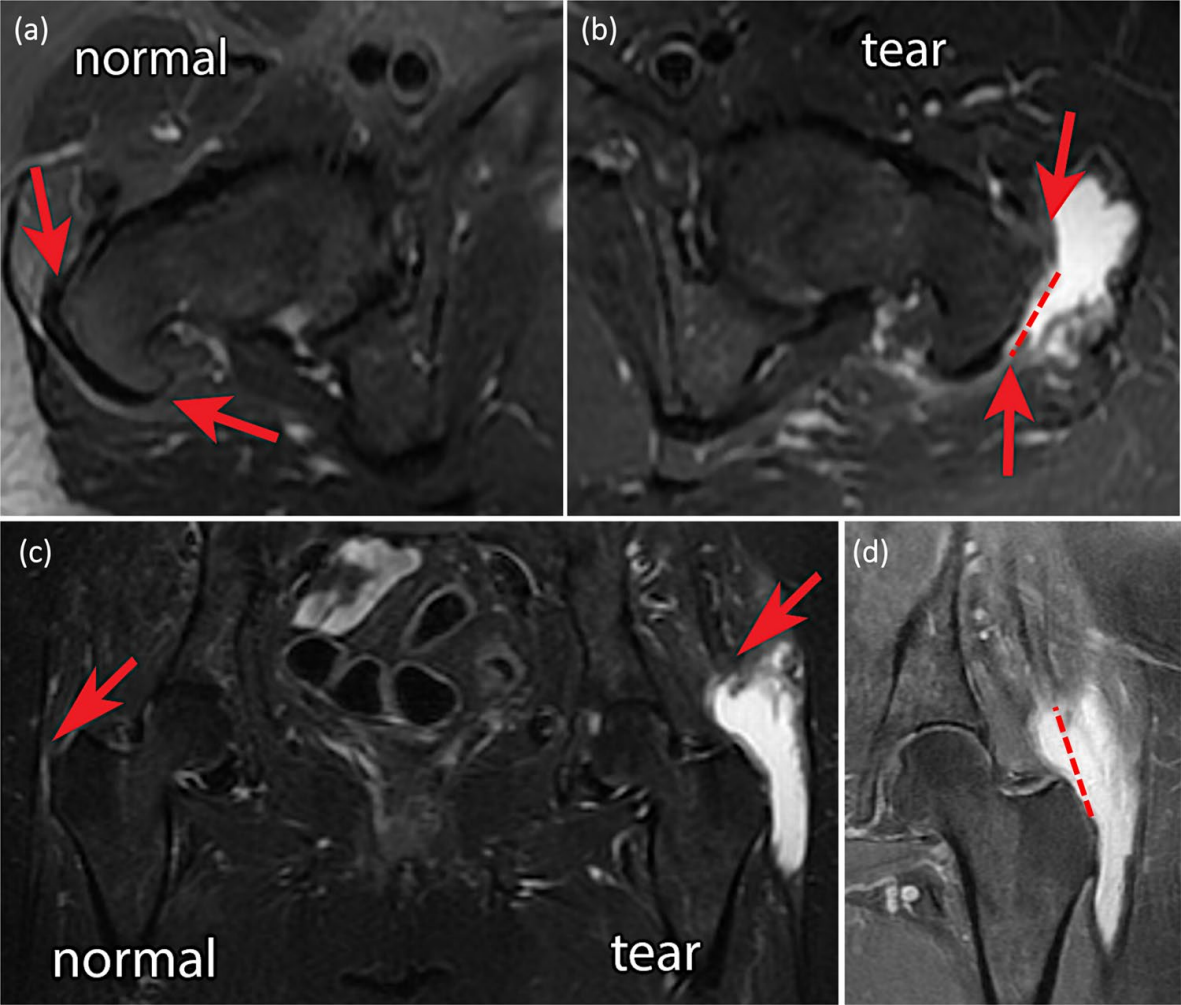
a–d Preoperative MRI revealing a GTT on the left side. In the axial views a the right healthy side and b the anteroposterior detachment on the lateral aspect of trochanter. In the coronal plane c and d the retraction of the torn gluteal muscle compared to the healthy contralateral hip
